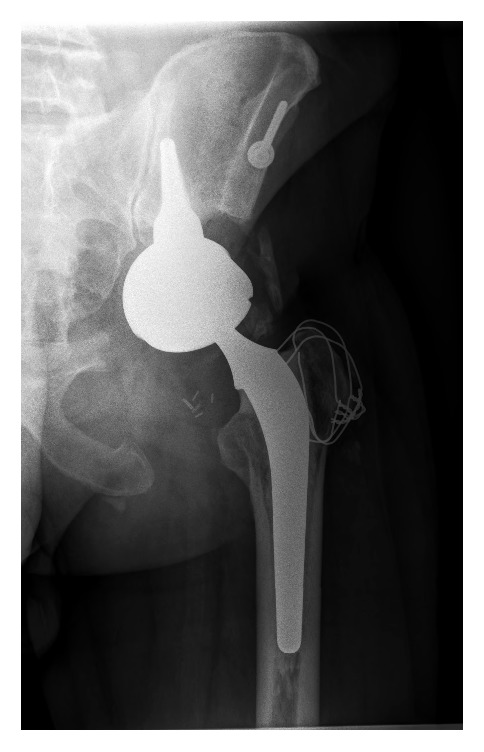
Radiographs: 12 months after surgery.
